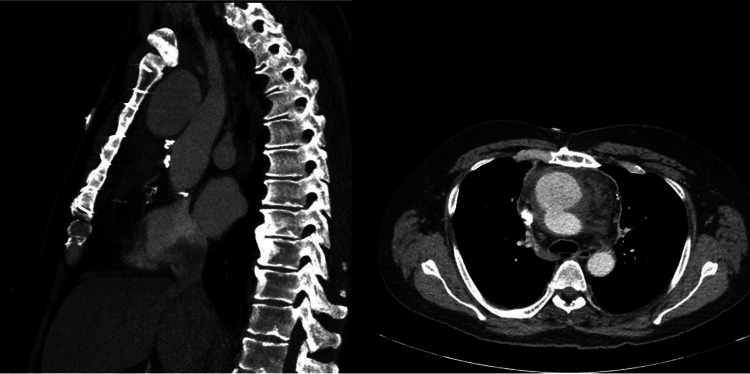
The follow-up computed tomography angiography with and without contrast demonstrating significant enlargement of the pseudoaneurysm arising from the anterior margin.
